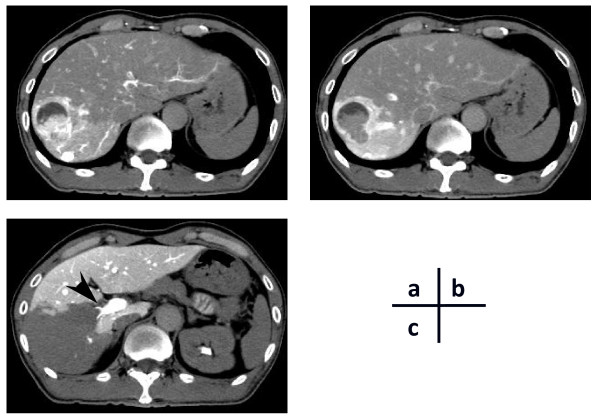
Computed tomography arteriography (CTA) of the liver tumor in the early phase (A) and the delayed phase (B). C) Computed tomography during arterial portography (CTAP) showing a portal vein tumor thrombus (arrow) and a perfusion defect in the entire right lobe.
